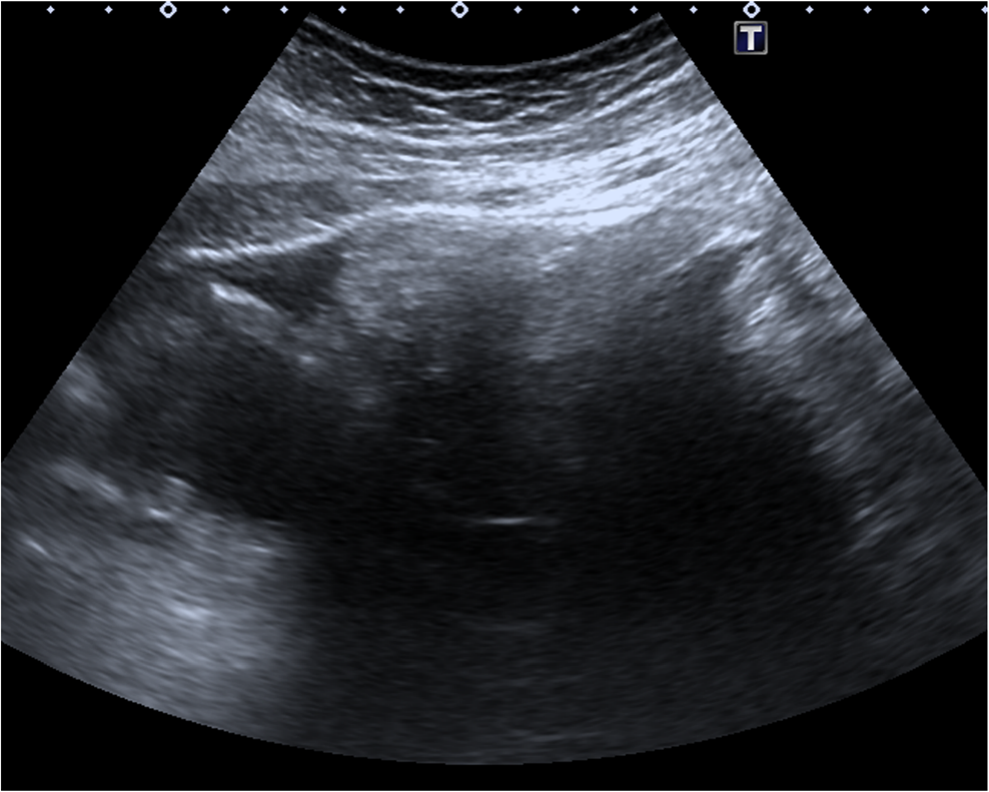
Transverse sonogram of a 16-year-old female with a mature cystic teratoma. An amorphous echogenic region is seen in the near field that causes posterior shadowing and obscures the posterior portion of the lesion and any structures behind it
